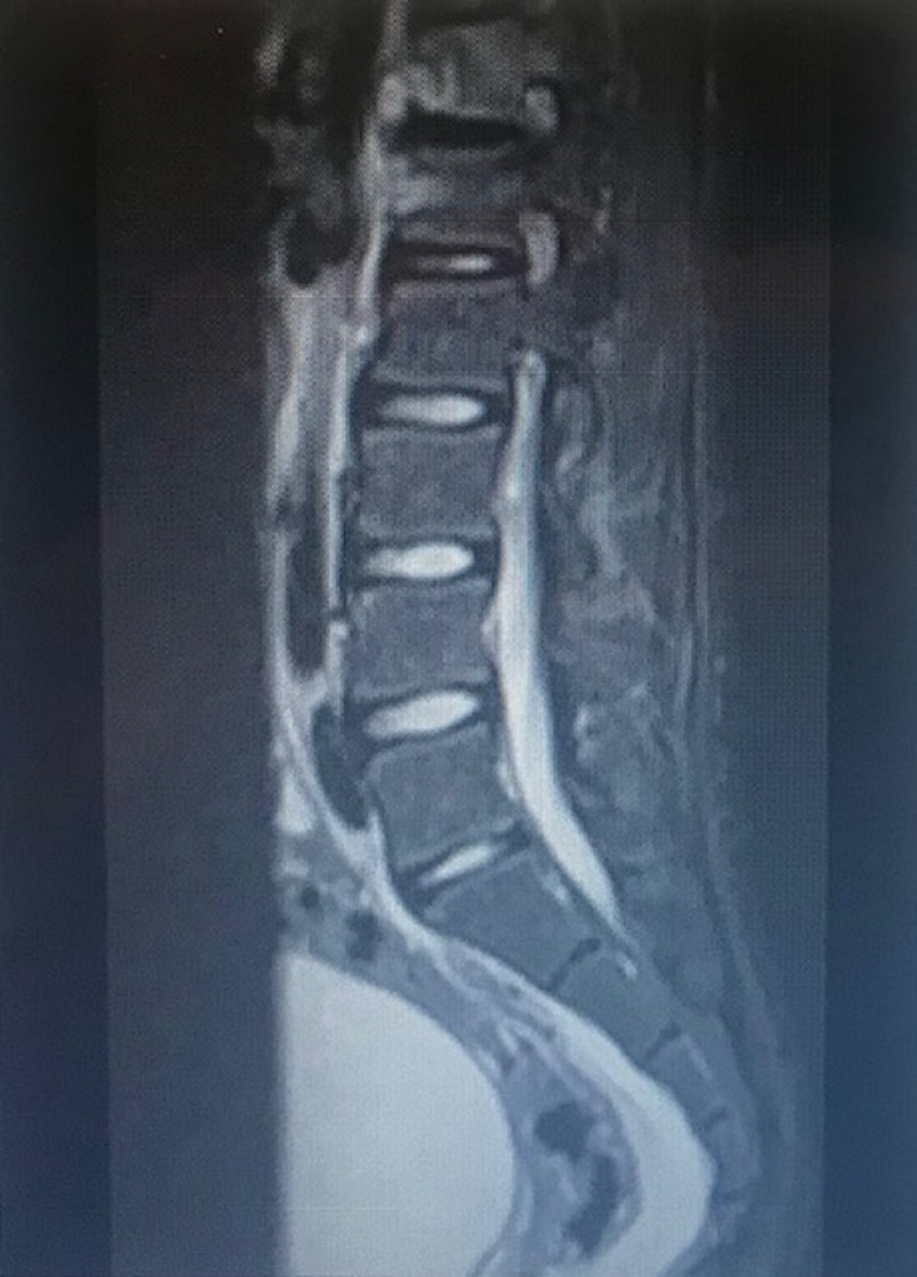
MRI of the lumbar spine of a 14-year-old child with spondylodiscitis involving the T12 and L1 vertebras and disc MRI, Magnetic resonance imaging.
